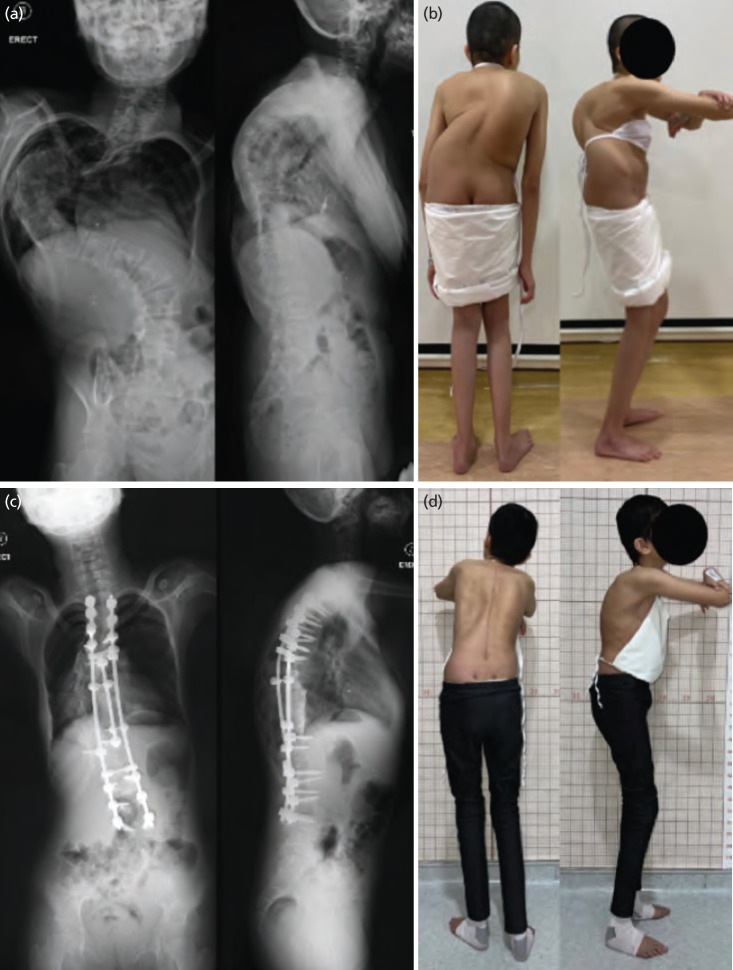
(a) Anterior-posterior and lateral view of the erect spine plain radiograph showing a Cobb angle 158° and kyphotic angle 90°. (b) Clinical photographs of the patient before HGT and HPT in posteroanterior view showed neck tilt, right truncal shift with prominent thoracic hump and uneven shoulder level. The right sagittal view showed thoracic kyphosis malalignment. (c) Anterior-posterior and lateral view of the erect spine plain radiograph after posterior spinal fusion from T1 to L3, showing Cobb angle of 73° and kyphotic angle of 40°. (d) Clinical photographs of patient show improved scoliosis and thoracic kyphosis with height increase from 137cm to 153cm.
